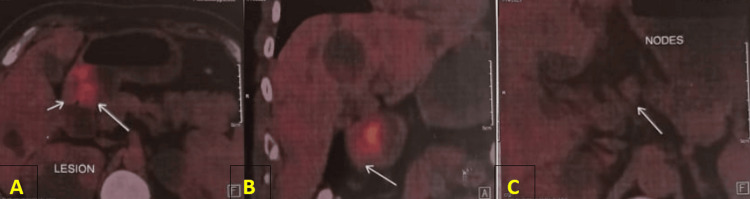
8-FDG PET scan images showing (A and B) asymmetric thickening in the antropyloric region with (C) weakly metabolic regional nodes. 8-FDG PET: 8-Fluorodeoxyglucose positron emission tomography
